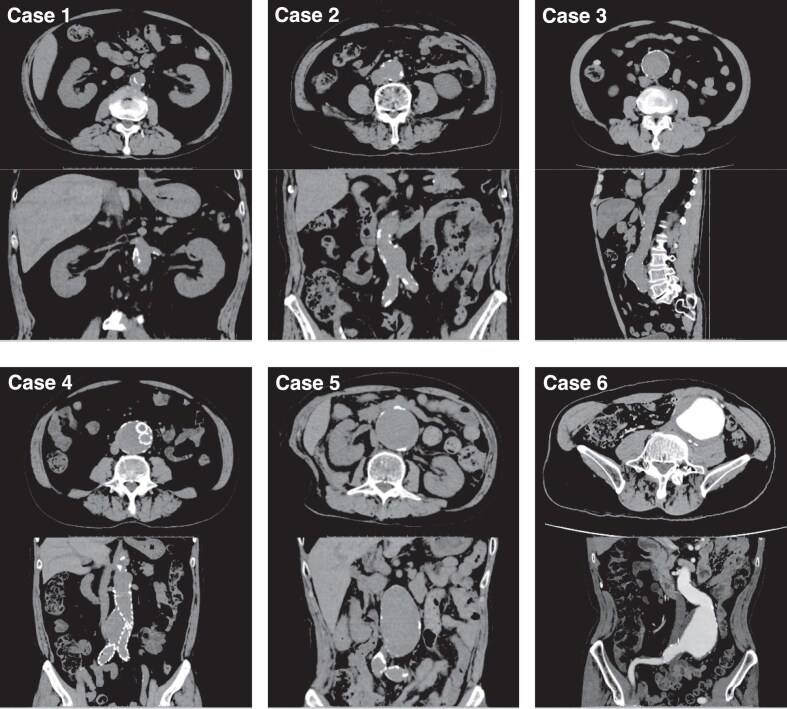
CT ima:ges of the patients with AAAs (patients 1–6).
